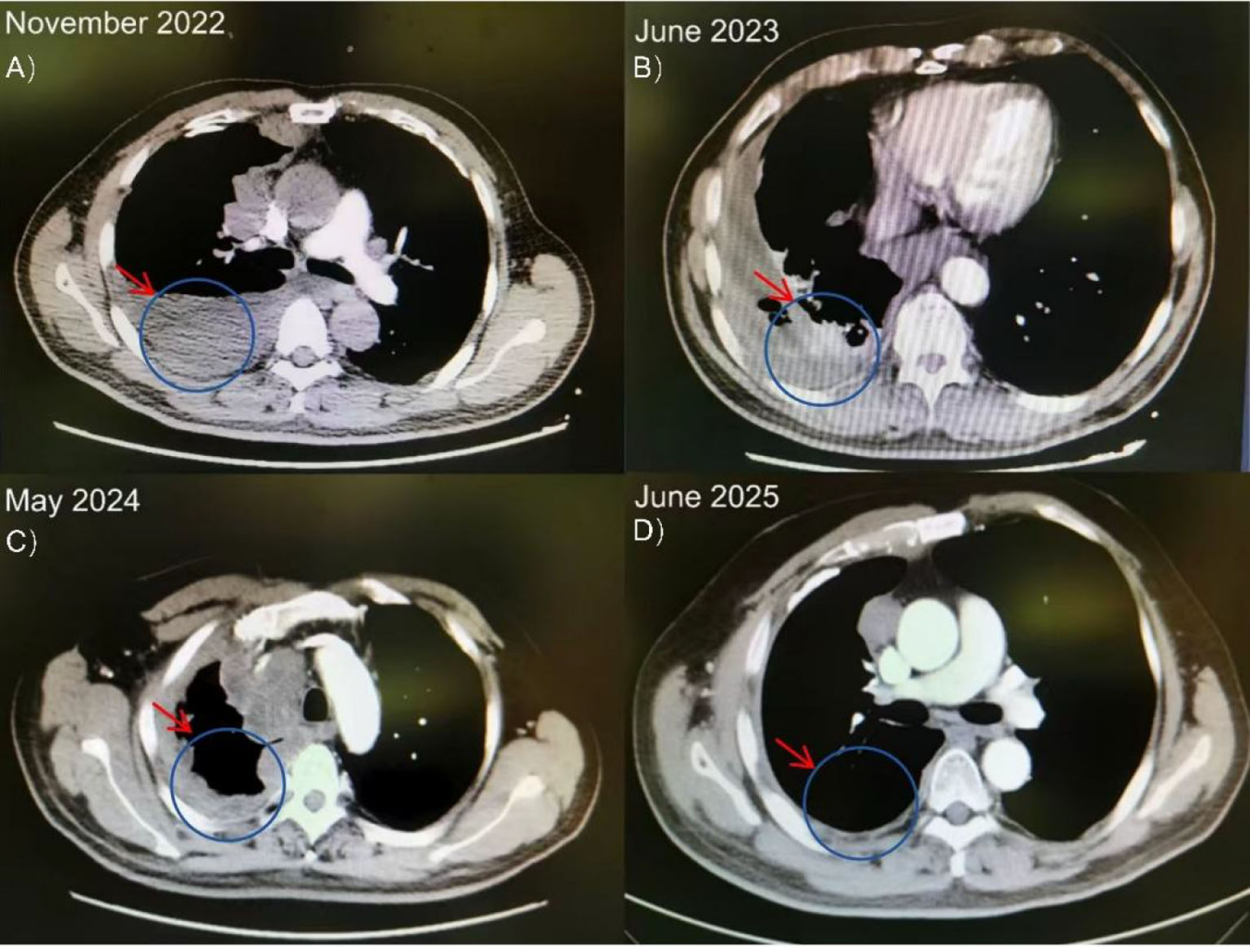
Serial axial chest CT scans of the patient with MPM. (A, B) Baseline imaging shows right pleural masses and effusion. (C, D) Subsequent scans demonstrate disease progression with the emergence of a new chest wall soft tissue mass (arrow) and increased pleural effusion despite second-line chemotherapy.
